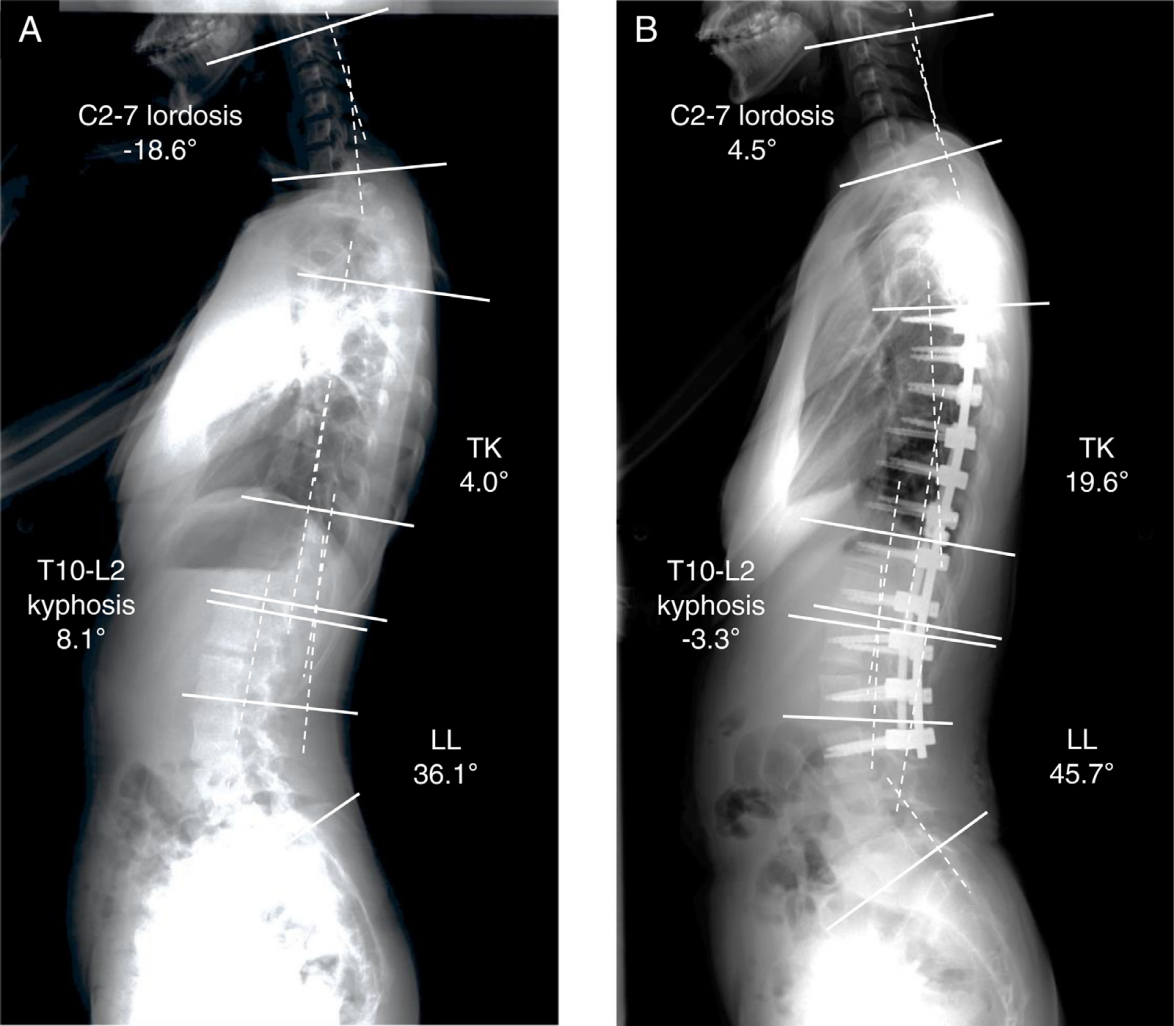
Representative pre- and 3-year postoperative whole spine standing sagittal radiographs for the patients with Lenke type 6 adolescent idiopathic scoliosis. After selective posterior correction and fusion surgery with pedicle screws from T5 to L3, each of the spinal sagittal alignments were corrected at a 3-year follow-up period. Preoperative C2-7 lordosis (C2-7 Cobb angle) of -18.6°, TK of 4.0°, T10-L2 kyphosis of 8.1°, and LL of 36.1°. Whereas, at 3-year post-operation, C2-7 lordosis of 4.5°, TK of 19.6°, T10-L2 kyphosis of -3.3°, and LL of 45.7°.
